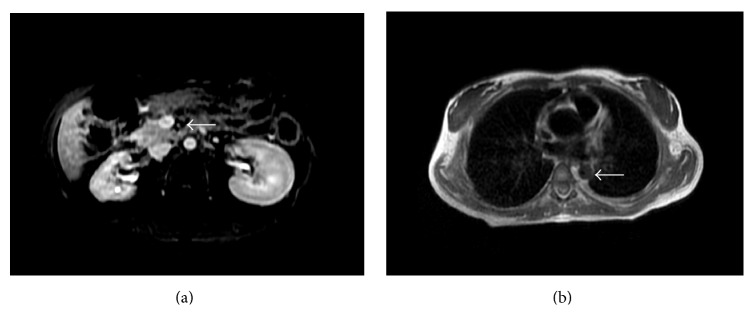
MRI and MRA of the abdomen and pelvis were obtained. (a) LAVA postcontrast sequence demonstrates mural thickening and marked luminal narrowing of the superior mesenteric artery. (b) Axial double inversion recovery sequence demonstrates increased focal narrowing of the descending thoracic aorta, measuring 1.6 cm in diameter. The white arrow represents in (a) SMA and in (b) descending thoracic aorta.
